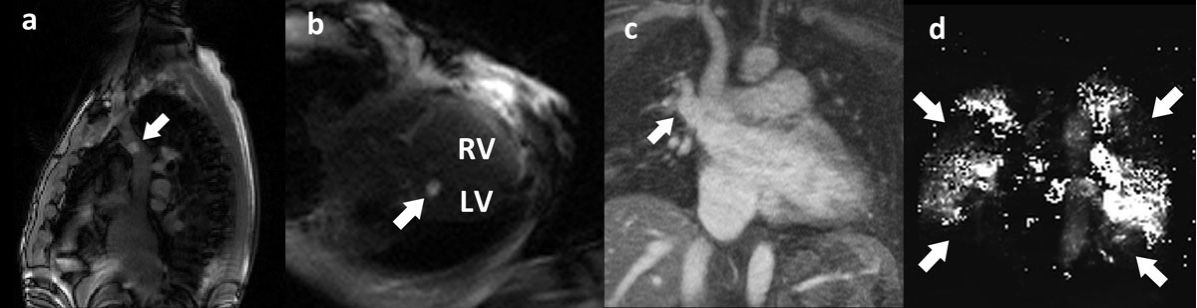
MRI catheterization images (a) Gadolinium-filled balloon-tip (arrow) catheter in superior vena cava. (b) Gadolinium-filled balloon-tip (arrow) catheter in left ventricle through atrial septal defect. (c) Anomalous pulmonary vein (arrow) draining into superior vena cava. (d) Lung perfusion map showing multiple filling defects (arrows).
